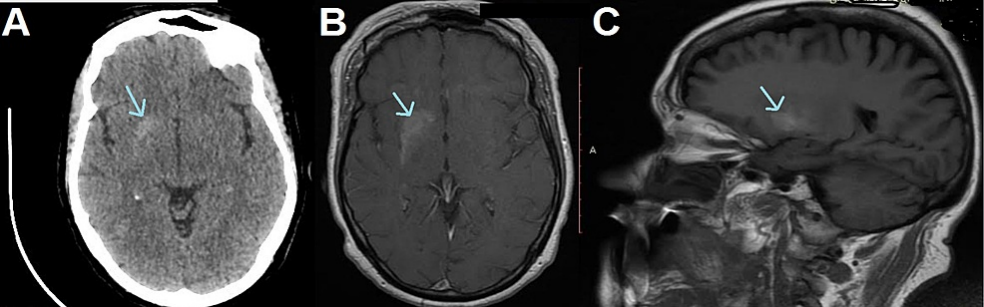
(A) Brain CT axial imaging showing hyperdensity of the right globus pallidum. (B, C) axial and sagittal MRI showing a hyperintense T1-weighted lesion in the striatal region.
